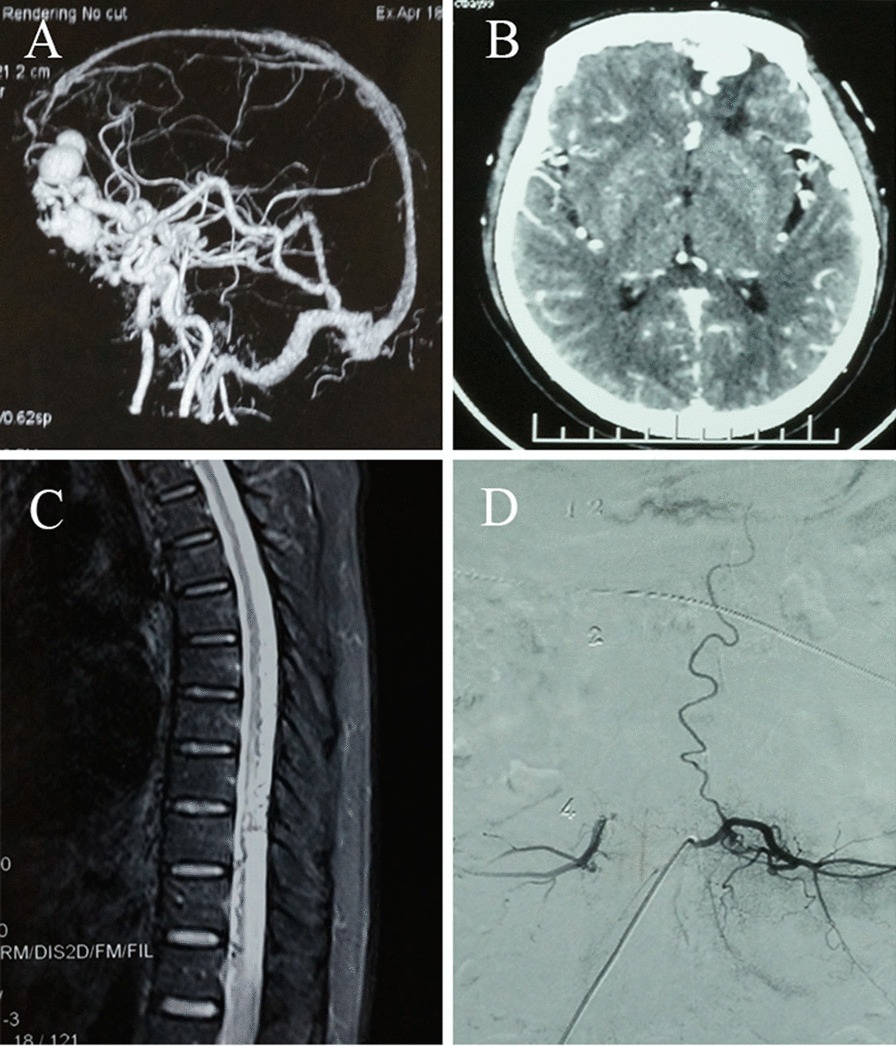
The imaging results of the dural arteriovenous fistula and the spinal dural arteriovenous fistula. A Computed tomographic angiography examination of the head shows a space-occupying venous bulb at the frontal base. B The axial computed tomographic angiography displays the bottom enhancement of the forehead. C Vermiform vascular shadows can be seen in the lower thoracic spinal cord. D Digital substraction angiography indicates a thick tortuous upward draining vein
